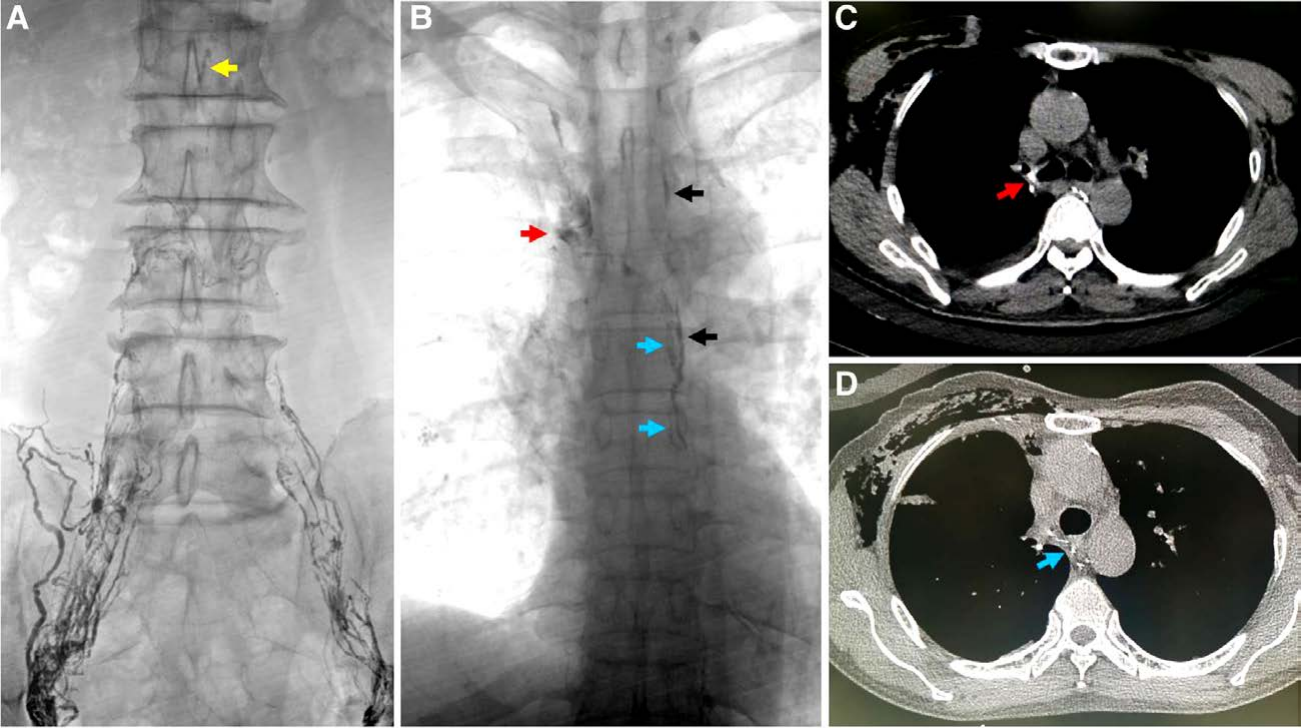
Lipiodol-based lymphangiography and CT showing an additional thoracic duct and leakage from the abnormal vessels. The additional lymphatic channel paralleled to the thoracic duct. Chylous leakage was found at the right pulmonary hilum. (A) Abdominal lymphatic channels. (B) Thoracic lymphatic channels. (C) Chylous leakage at the right pulmonary hilum. (D) Additional thoracic duct. (yellow arrow: chylous cistern; red arrow: leakage site; black arrow: thoracic duct; blue arrow: additional thoracic duct). CT = computed tomography.
